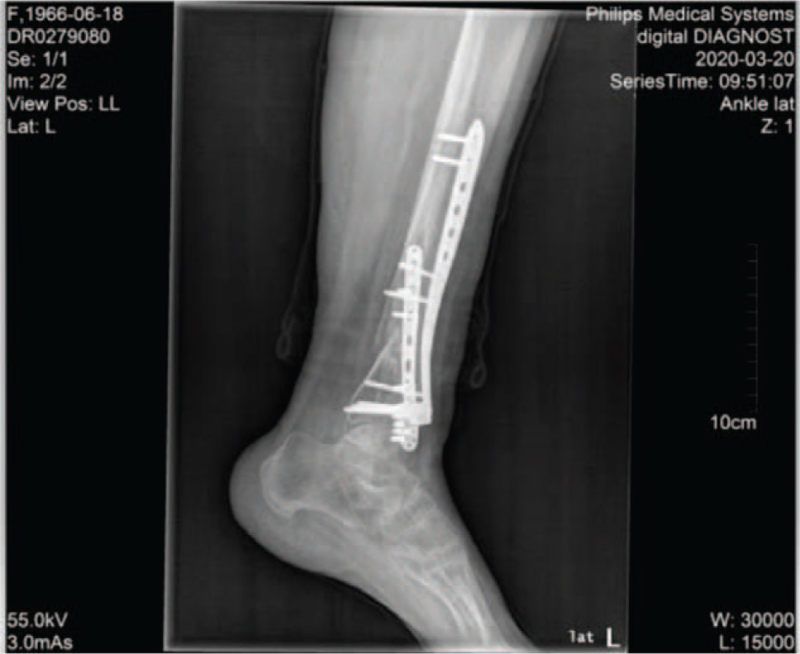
X-ray of fracture of the distal left tibiofibula.
